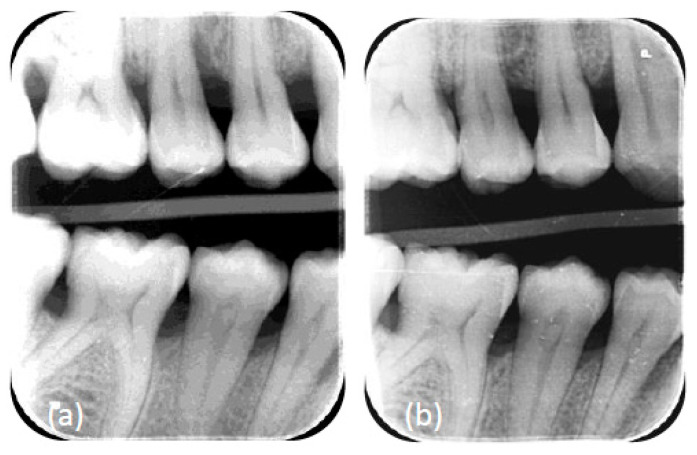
(a) Radiographic view of the angular defect 15 M before regenerative intervention. (b) Radiographic view of the angular defect mesial to 15 6 months after the intervention, attesting to a good healing capacity after periodontal surgery.
